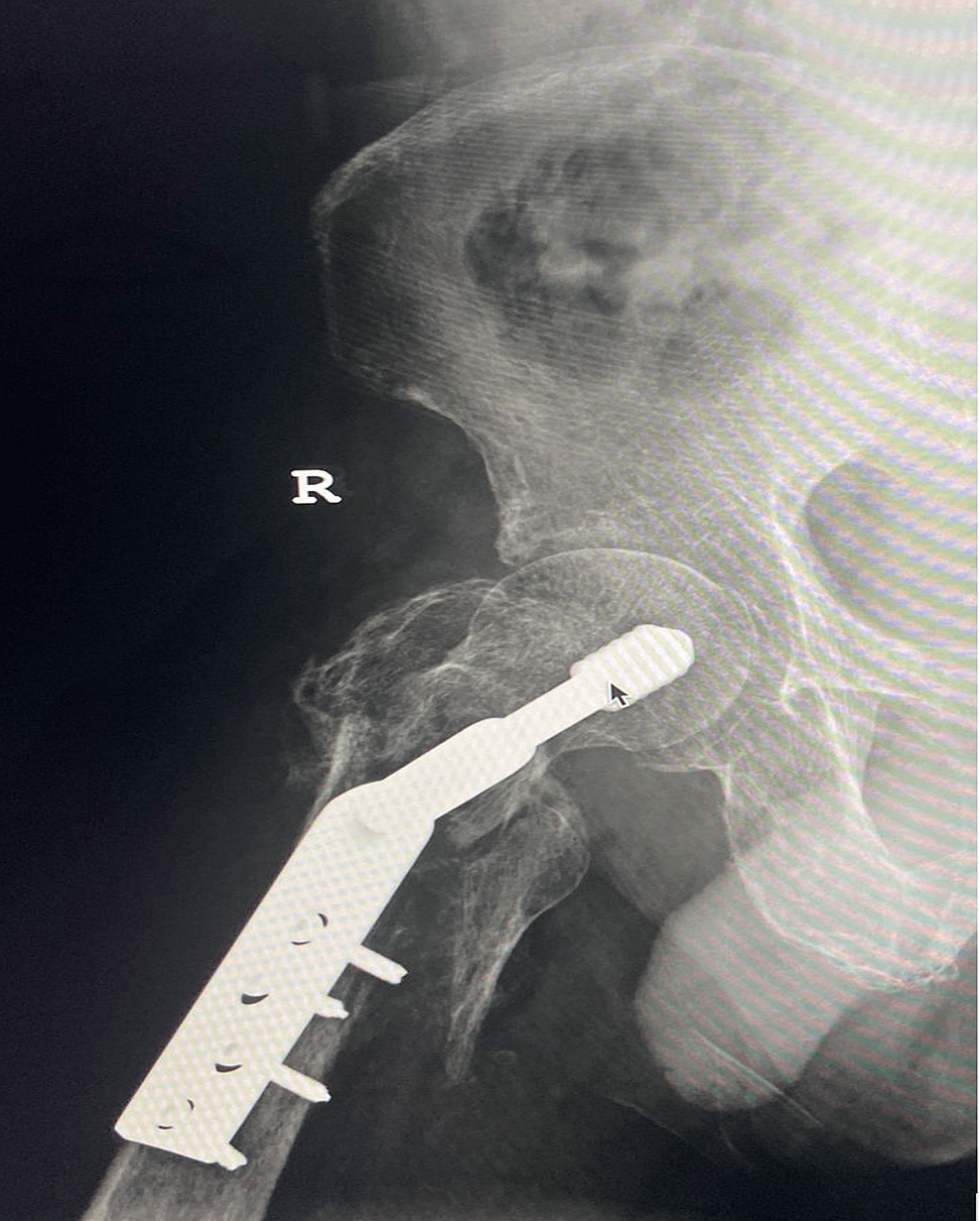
Anteroposterior radiograph of the right hip in extreme external rotation showing an unacceptable fracture reduction.
